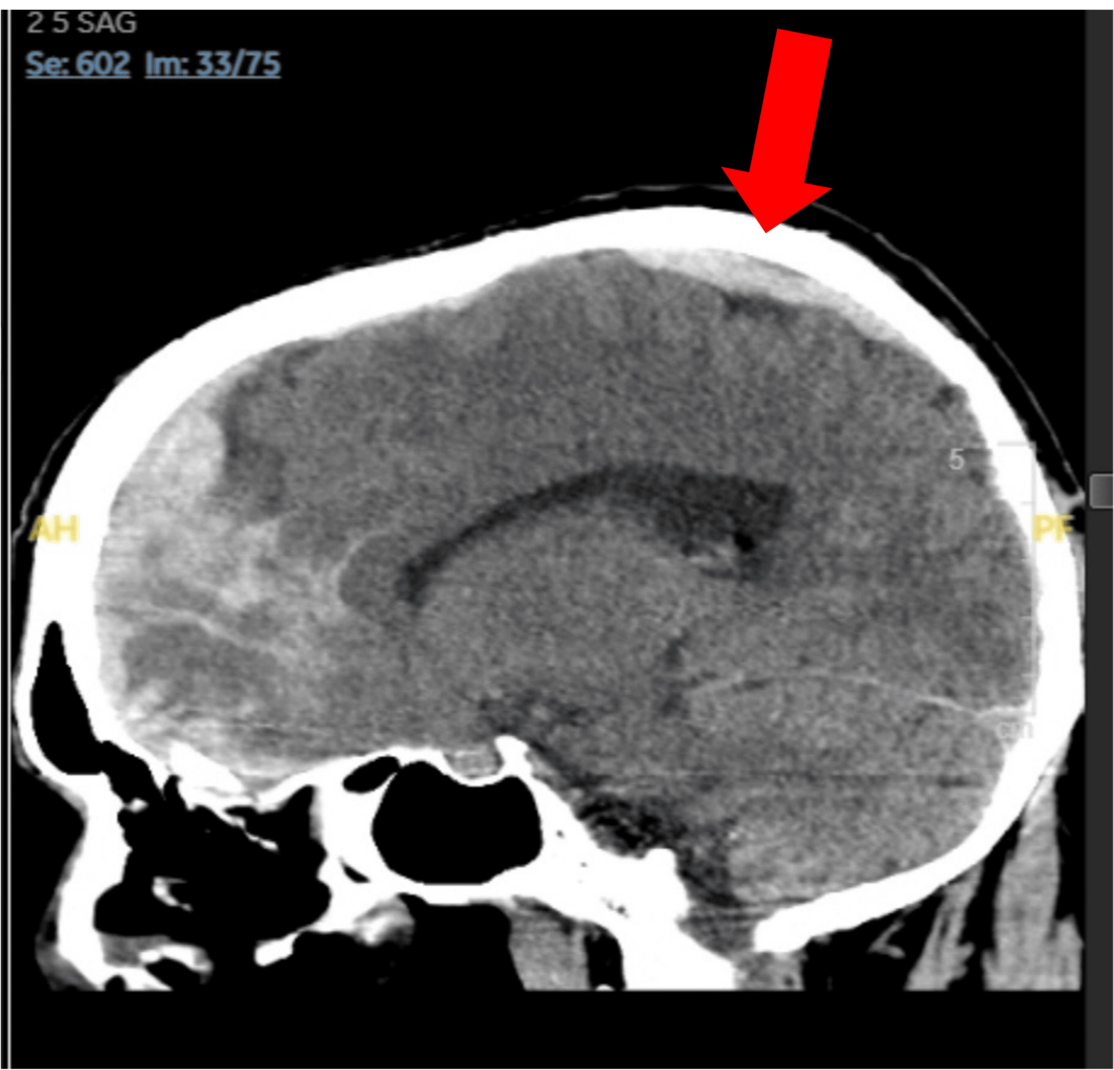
A CT scan of the brain without contrast, six hours after the initial CT, displaying worsening subarachnoid hemorrhage with developing intraparenchymal hemorrhage
